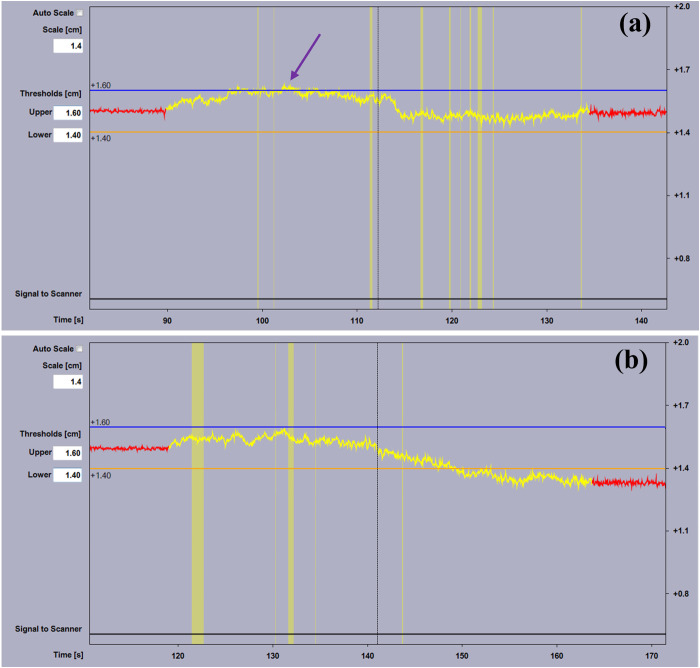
Comparison of two different calibration with weight for a scan length of 1 m. (a) The extended calibration proposed by Park et al. [18]; (b) The proposed calibration method. The yellow segment indicates the CT beam-on time. The purple arrow indicates the DIBH trace exceeding the predetermined thresholds during scanning.
